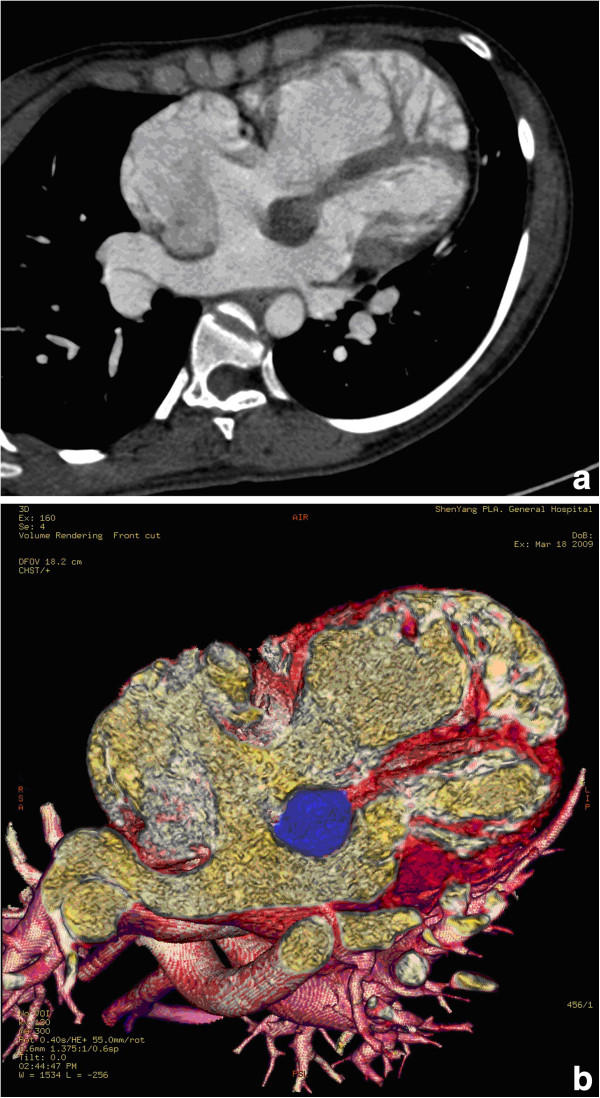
a,b Chest CT showing a 2.5 cm ASD, a 2.0 × 1.9 cm mass projecting into the left atrium, the average attenuation value was approximately 15 Hu.
